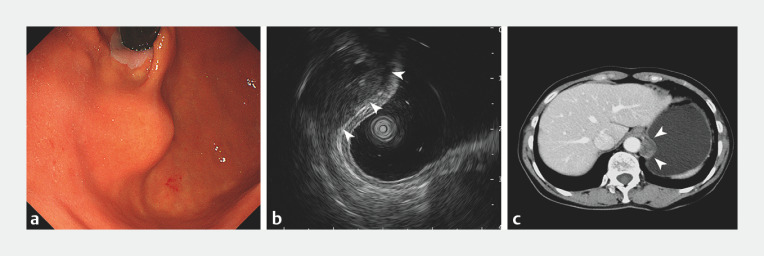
Endoscopic, endosonographic, and computed tomography (CT) findings of fundal submucosal lesions.
